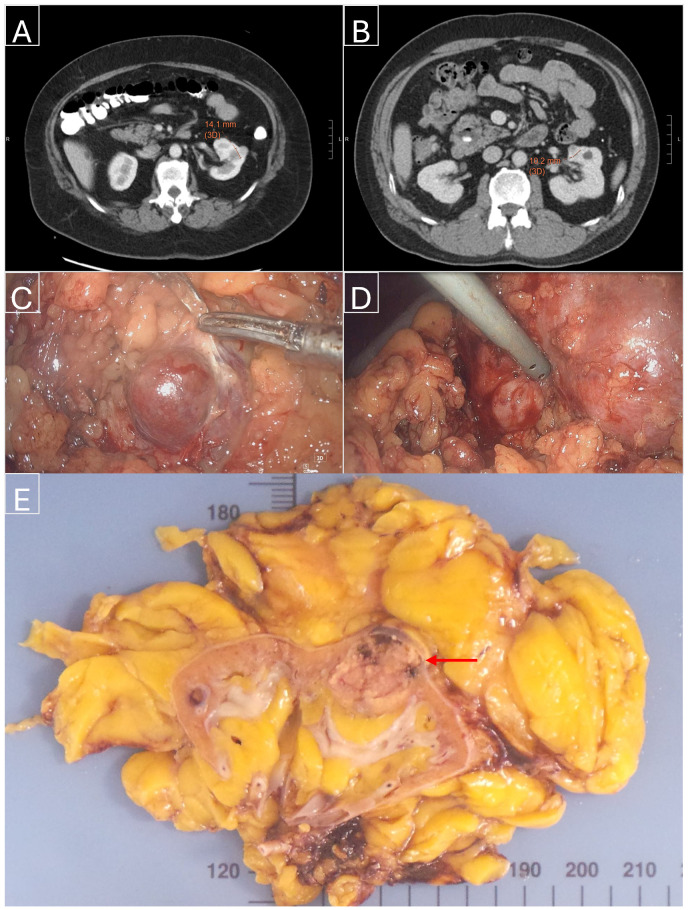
Contrast-enhanced CT, intraoperative and gross images of selected patients. (A, B) Contrast-enhanced CT revealed a 1.4 cm tumour in the middle pole of the left kidney in patient 1 and a 1.0 cm lesion in the anterior middle pole of the left kidney in patient 2, respectively. (C, D) Intraoperatively, a well-circumscribed cystic tumour was found at the lateral side of the left kidney in patient 1 and near the hilar region of the left kidney in patient 2, respectively. (E) A nodular tumour (arrow) was identified in the middle pole of the right native kidney of patient 4, who was diagnosed with lupus nephritis and end-stage kidney disease.
